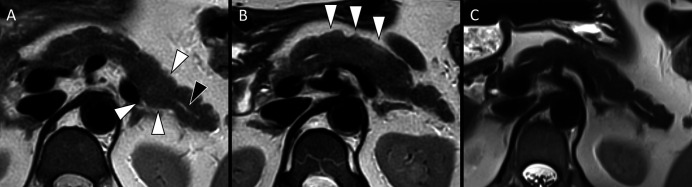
Serial T2-weighted magnetic resonance images of the pancreas. (A) Initial MRI (February 2021) demonstrates focal enlargement and T2 signal hyperintensity at the junction of the pancreatic body and tail (white arrowheads) with prominence of the upstream pancreatic duct (black arrowhead) suspicious for focal pancreatitis. (B) Follow-up MRI (March 2021) demonstrates worsening enlargement and signal abnormality of the pancreas, more diffusely involving the pancreatic body (white arrowheads). (C) Follow-up MRI (October 2021) demonstrates resolution of parenchymal changes with normal size and lobulated contour of the pancreas. MRI, magnetic resonance imaging.
